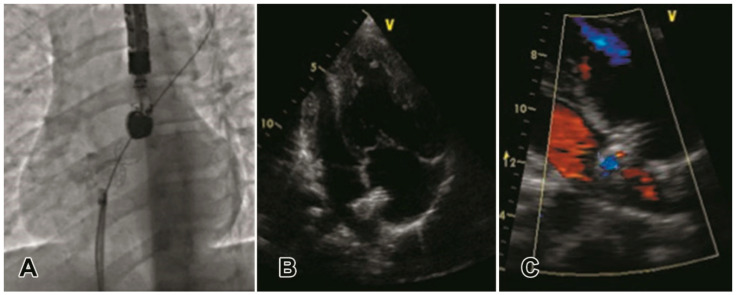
Examination after AS with stenting. (A—fluoroscopy immediately after stenting; B, C—transthoracic echocardiography at follow-up). Stent is located correctly. Right heart chambers are enlarged; right-to-left shunt across the atrial septum is visualized.
